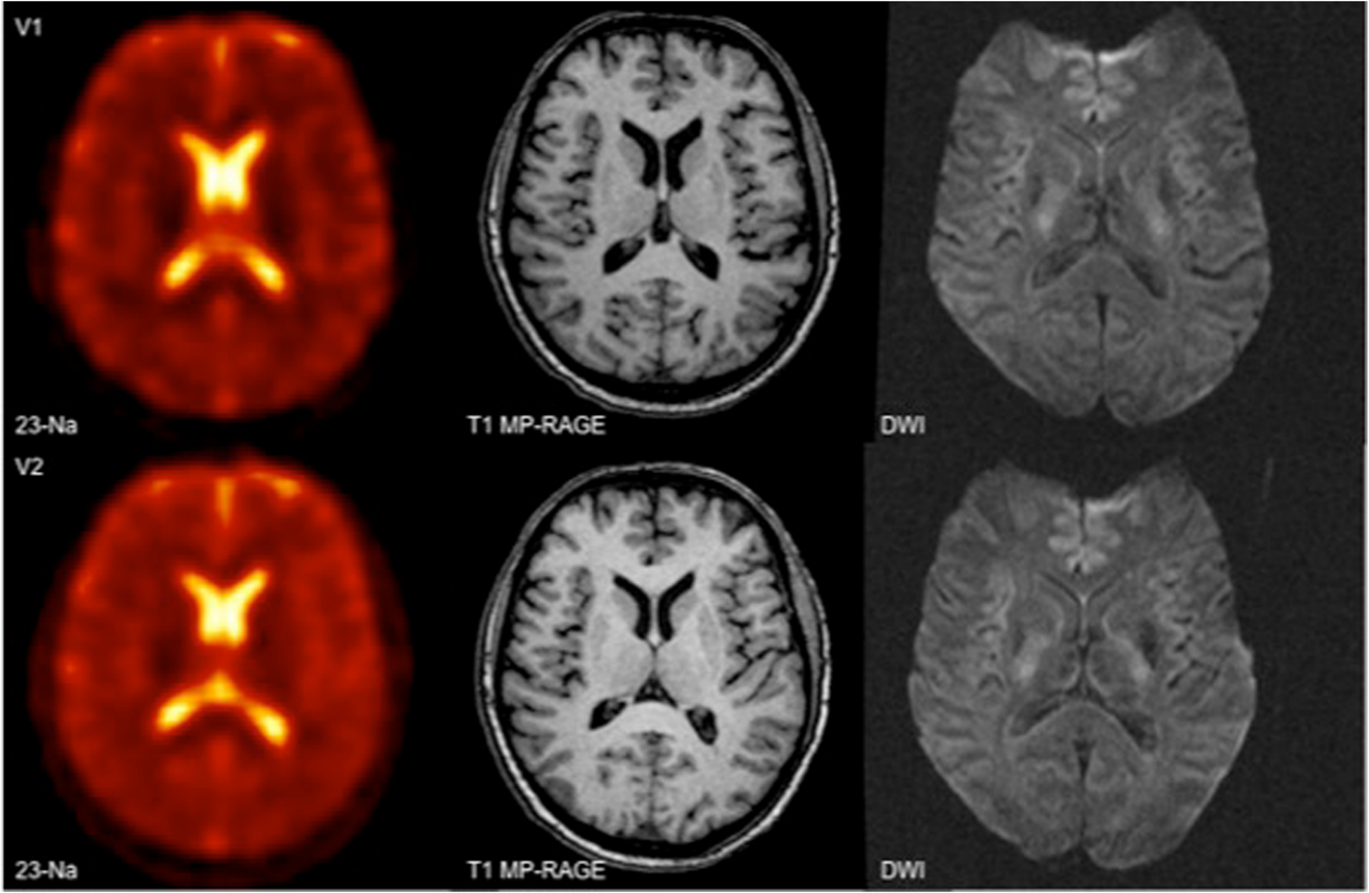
Top*: First examination (V1) on the first examination day with ^23^Na-image, T1 MP-RAGE and DWI (from left to right); *bottom*: Second examination (V2) on the first examination day
